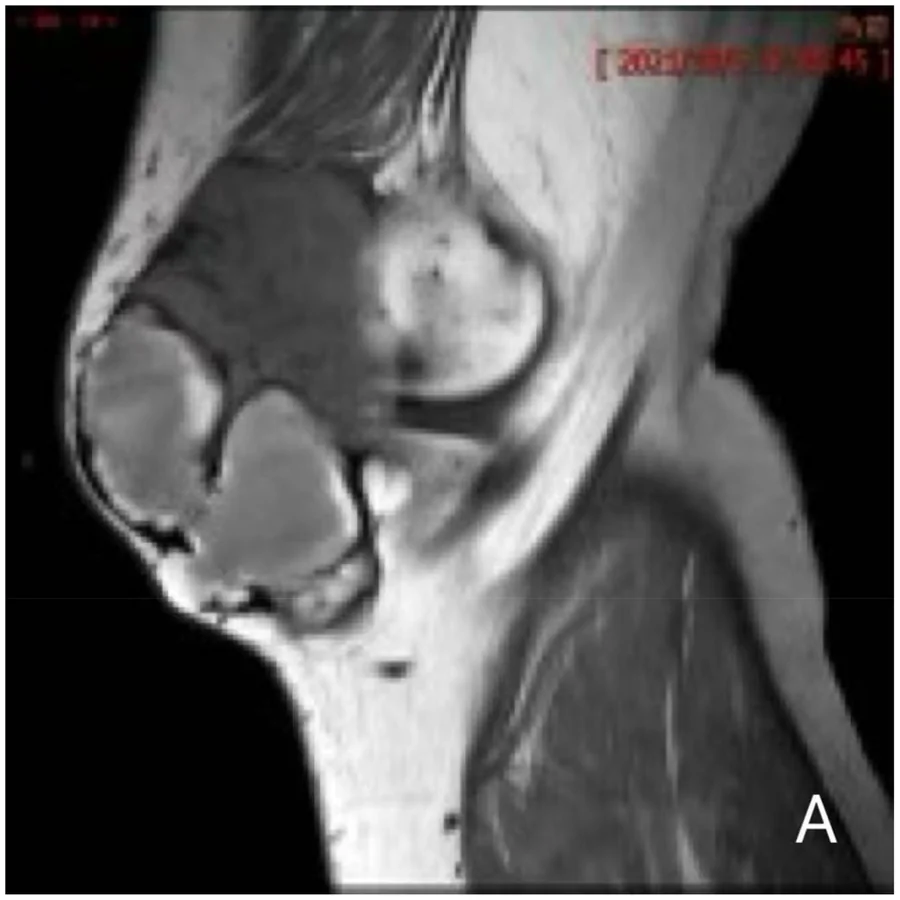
Preoperative MRI showed intra-articular extension of the lesion into the patellofemoral joint cavity.
